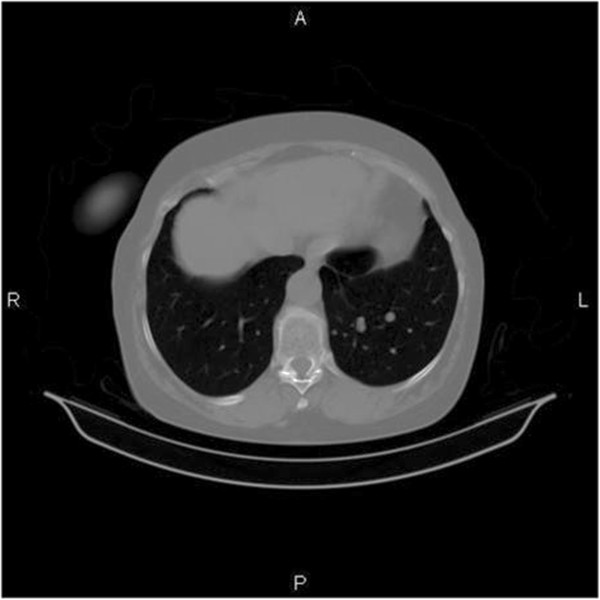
Chest computed tomography. The pulmonary nodular tumor invasion.
